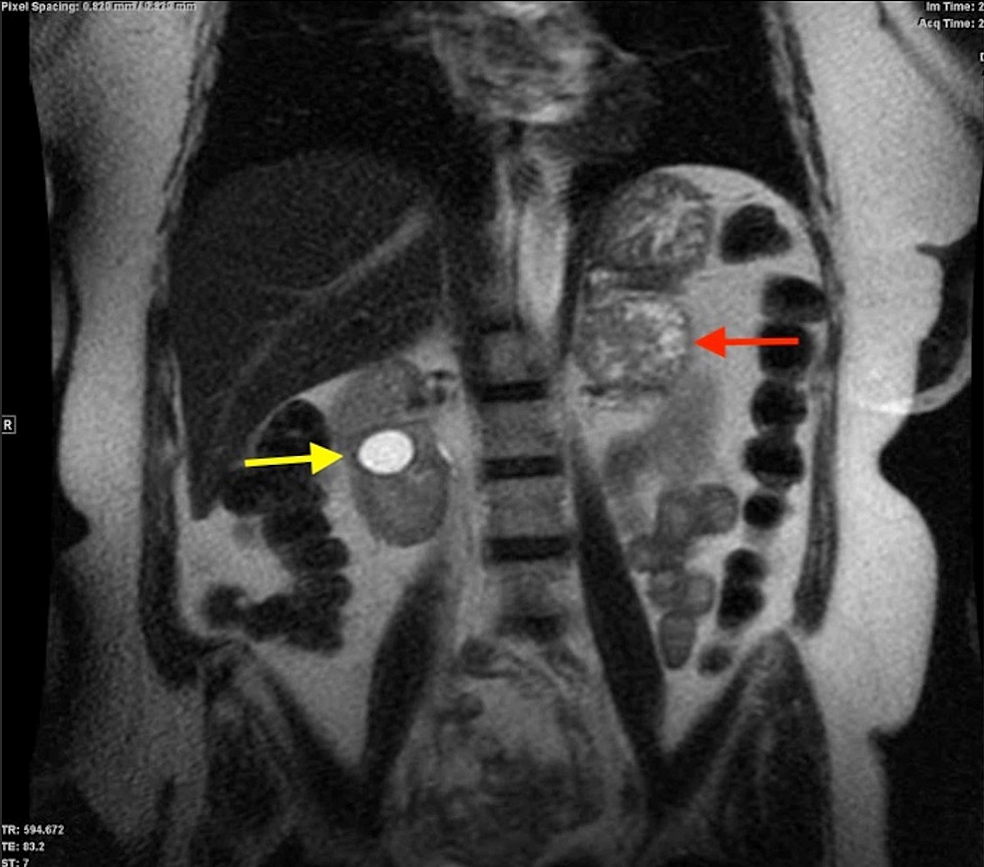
Coronal view- T2-weighted MRI showing a left adrenal mass (red arrow) and a right renal cyst (yellow arrow) MRI: magnetic resonance imaging
